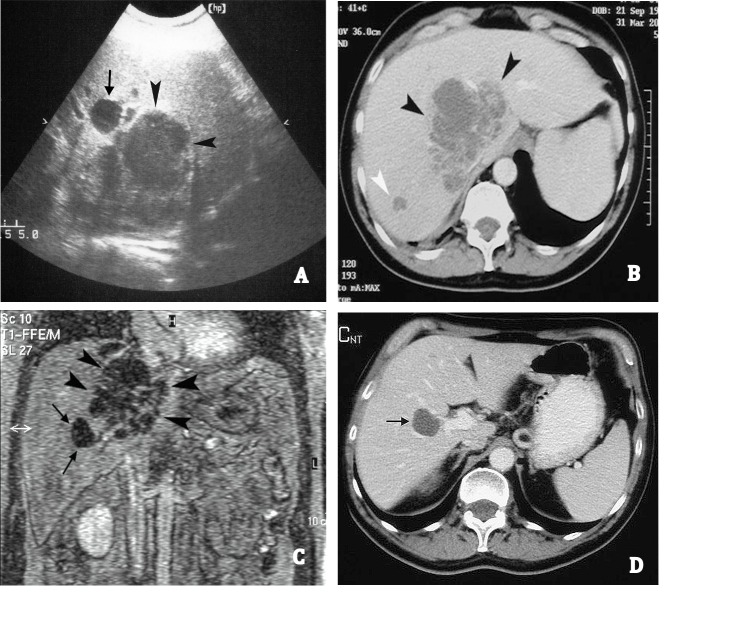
A. US shows a large hypoechoic lesion with ill-defined margins (black arrow heads) and an anechoic lesion (black arrow). B. Abdominal spiral CT reveals a hypointense area that was slightly enhanced in the periphery and in septations after contrast administration (black arrow heads) and a small lesion with similar density (white arrow head). C. MRI reveals a large sepimented lesion (black arrow heads) and a small hypointense lesion referable to a liver cyst (black arrows). D. A contrast-enhanced CT carried out 20 days after the discharge shows a hypointense lesion referred to a liver cyst (black arrow) and a complete resolution of the abscess.
